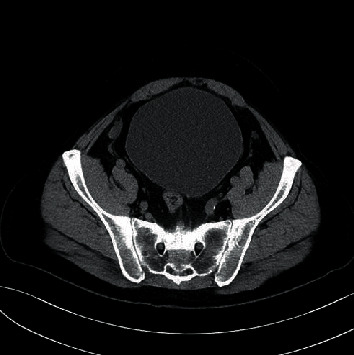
Noncontrast CT abdomen in transverse plane again demonstrates enlarged bladder with no appreciable surrounding enlarged lymph nodes.
